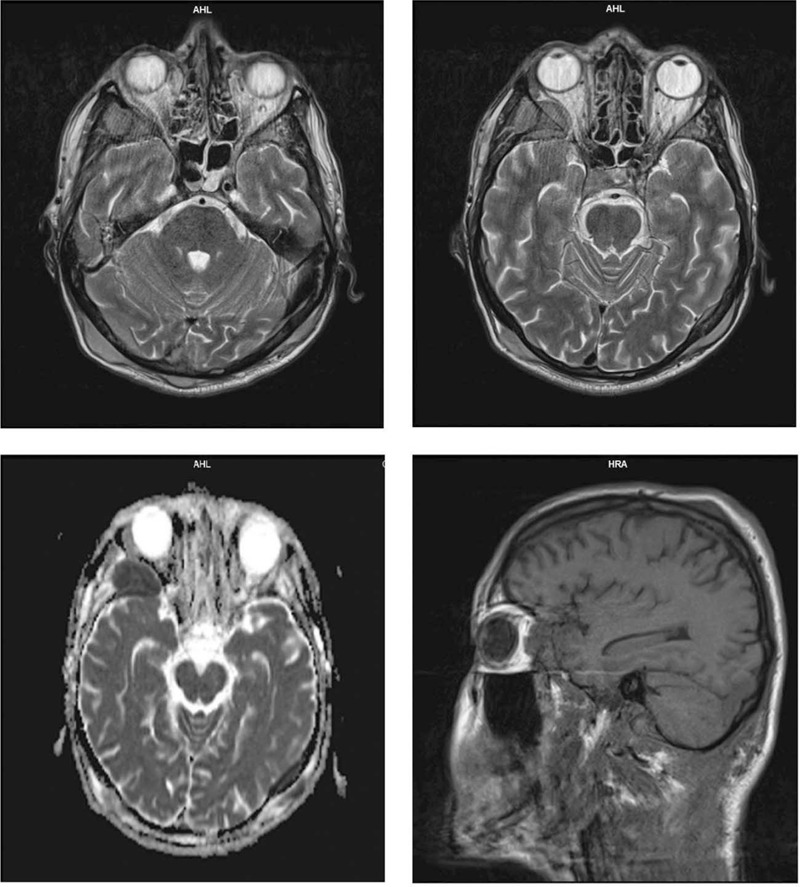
MRI sequences. MRI = magnetic resonance imaging.
